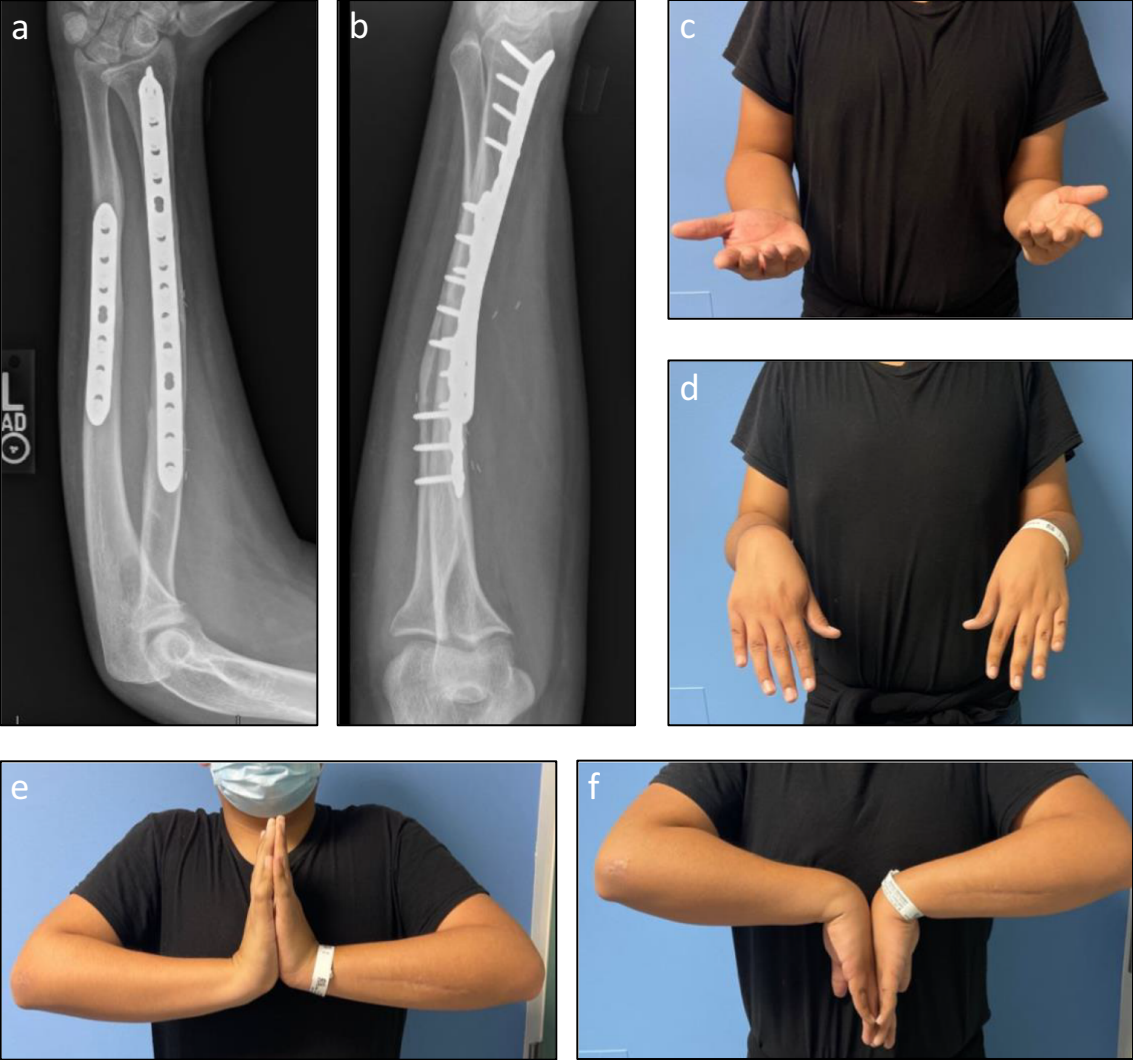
Seven months postoperative follow-up of patient in Fig. 2. a–b Orthogonal radiographs of corrected forearm with residual dorsal prominence of the distal ulna which is currently asymptomatic. c Maximal supination. d Maximal pronation. e Maximal wrist extension. f Maximal wrist flexion. (Courtesy of Sanjeev Sabharwal, MD, MPH)
